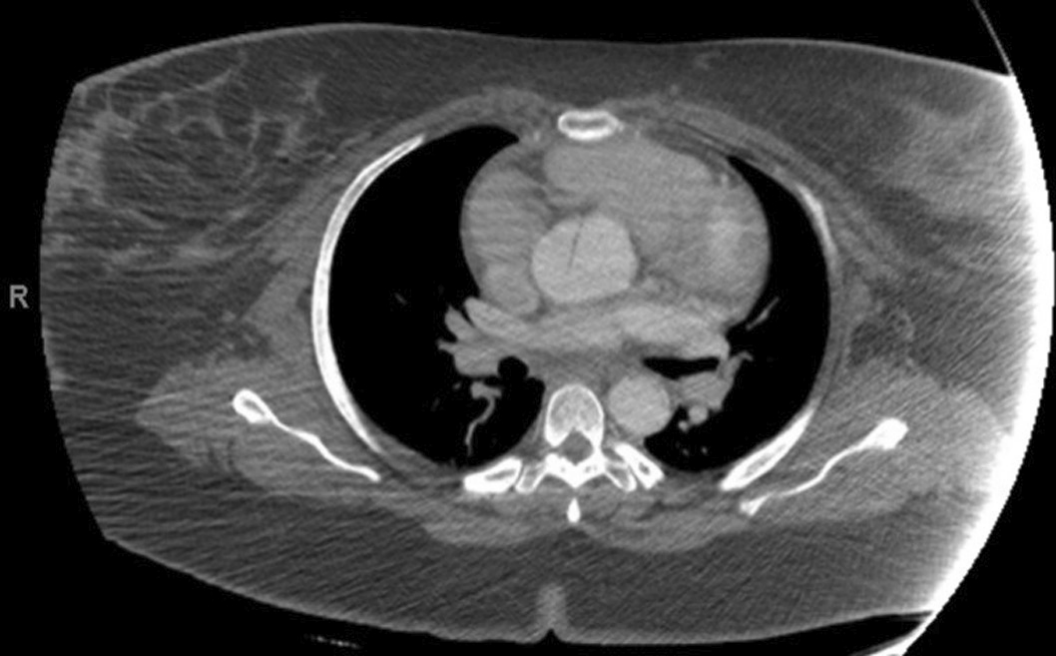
Initial computed tomography angiogram of the patient's chest obtained on hospital admission Day 3, showing a Stanford type A aortic dissection involving the ascending aorta.
